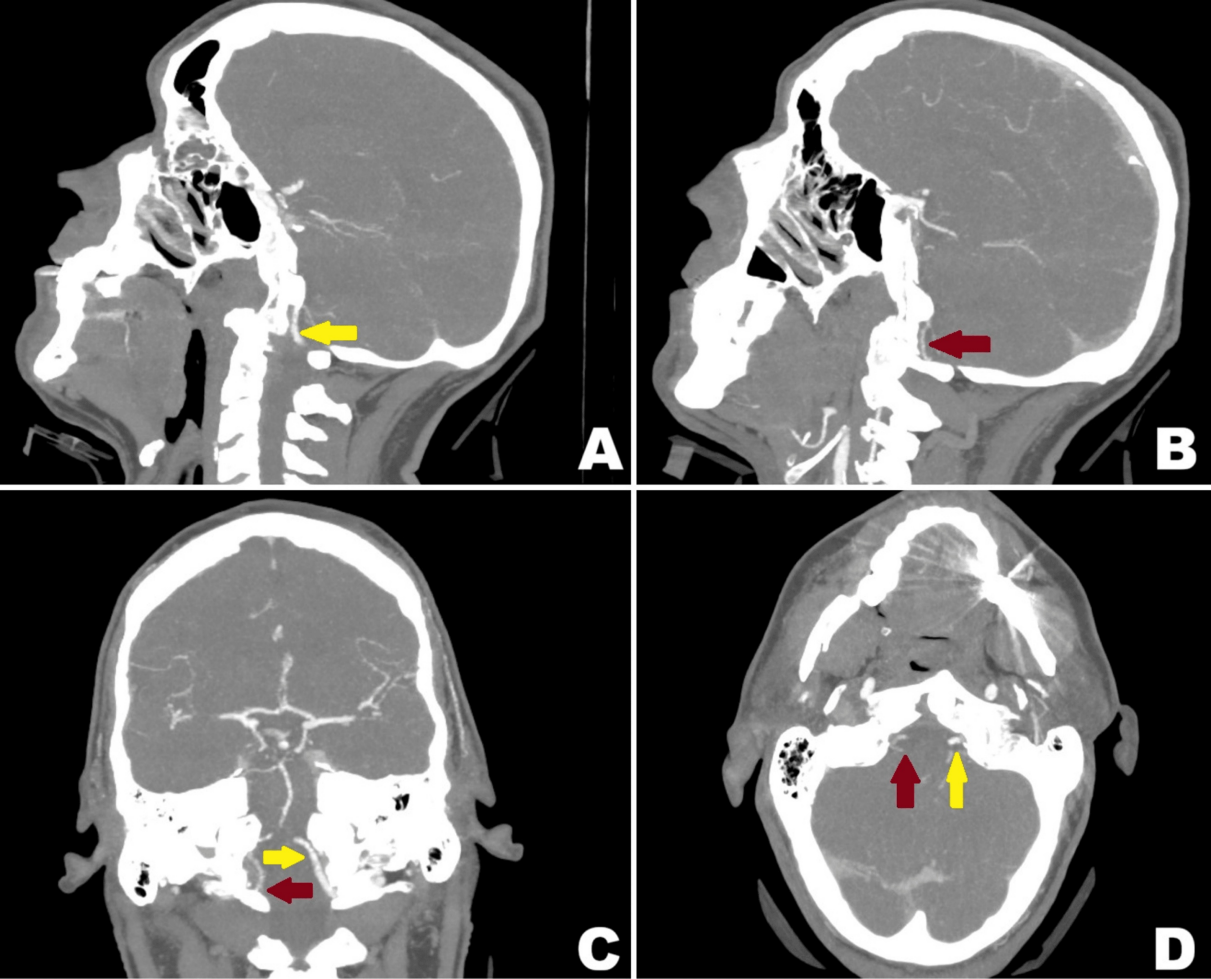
CT angiogram (vascular window) of the head and neck (A, B, C, D) showing subtle mural irregularity of the right vertebral artery (subfigure C, red arrow) at the level of the C1 fracture site. The right V4 segment appears diminutive (subfigure B, C and D, red arrows) compared to the left (subfigure A, C and D, yellow arrow), findings suggestive of a possible intimal injury. CT: computed tomography
